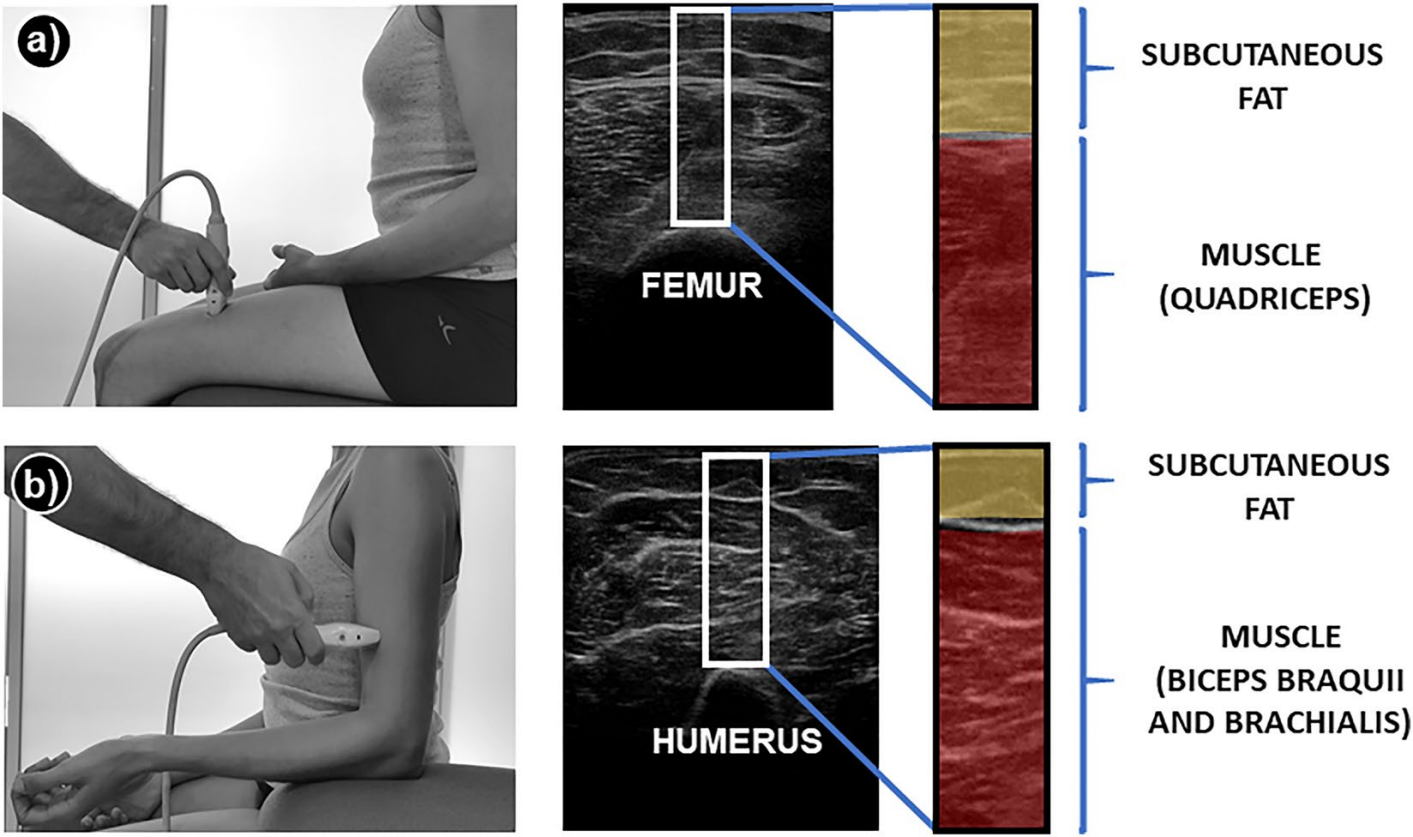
Position of the patient and ultrasound probe and range of interest (white rectangle) of sample images (a: quadriceps; b: biceps/brachialis).
